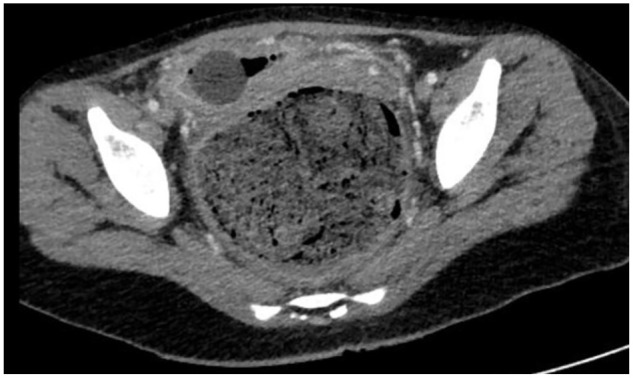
An axial CT showing an unchanged rectal fecaloma.
